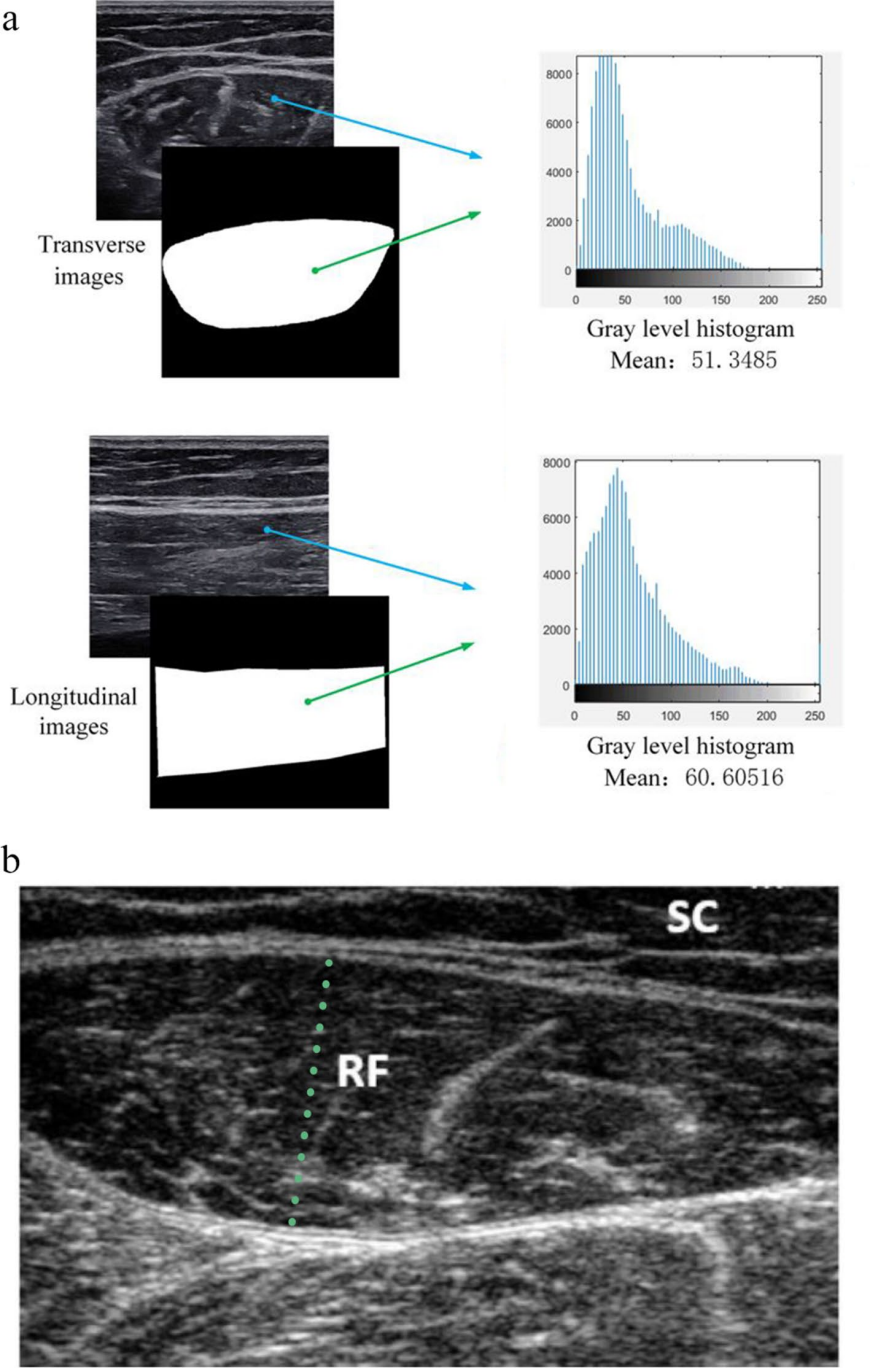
Ultrasound assessment of shear wave elastography and cross-sectional area (CSA). (a) The region of rectus femoris was selected in transverse and longitudinal images followed by the calculation with echo intensity analysis [27]; (b) CSA of the rectus femoris [35]. Copyright, *Oxford University Press* and *John Wiley and Sons*
